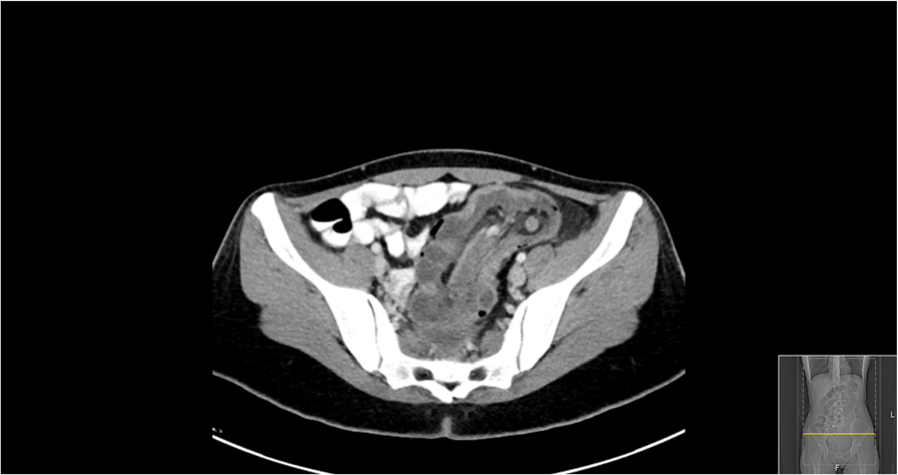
Axial section of pelvic CT revealing "sausage" sign of ileocolic intussusception to level of rectum.
